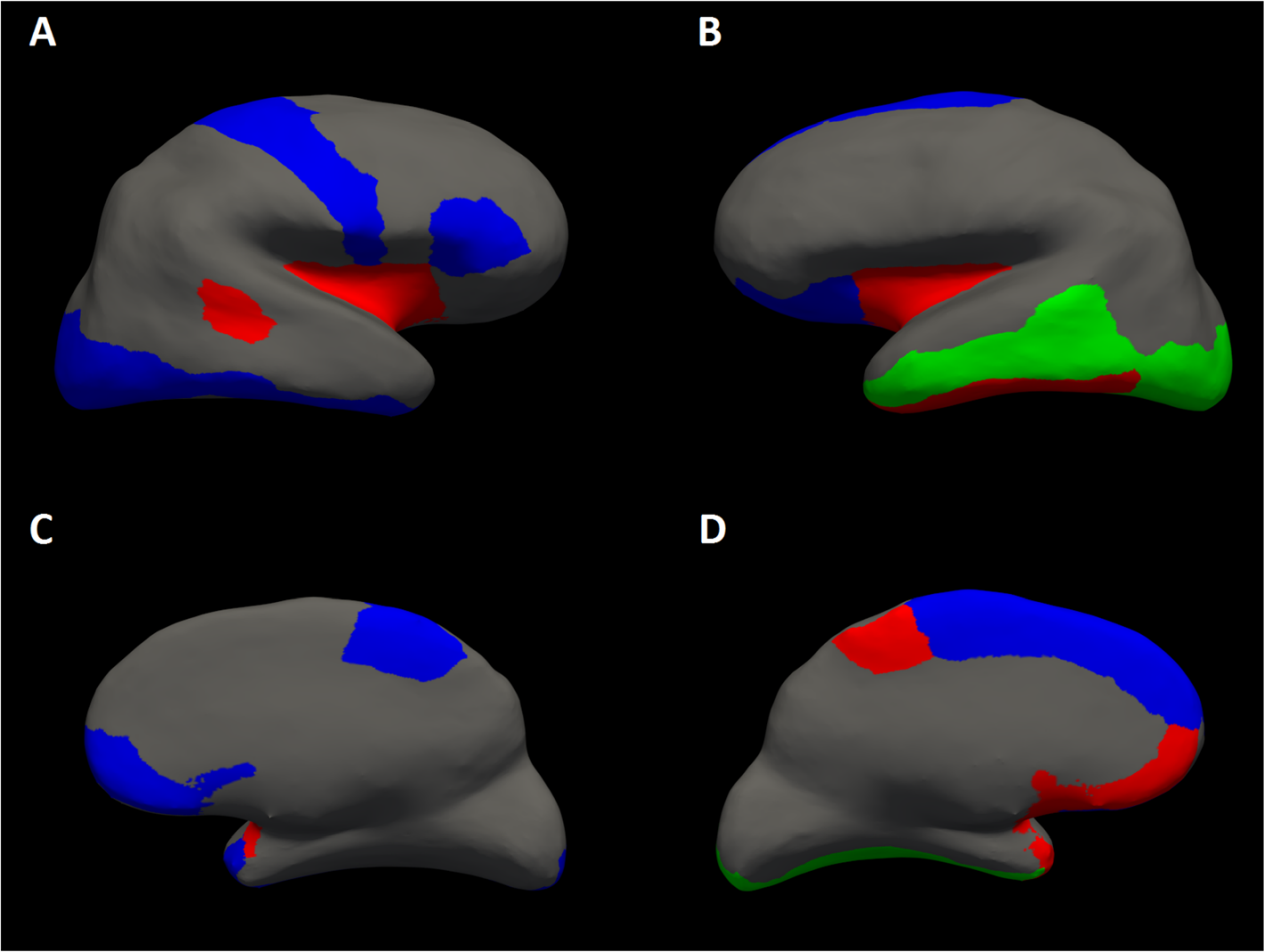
Statistically significant brain metabolic activity differences in ^18^FDG-PET (green), ^18^FDG-PET adjusted by brain structure (blue) or both analysis models (red) between healthy controls and chronic migraine. A Right hemisphere lateral view, (B) Left hemisphere lateral view, (C) Right hemisphere medial view, (D) Left hemisphere medial view
